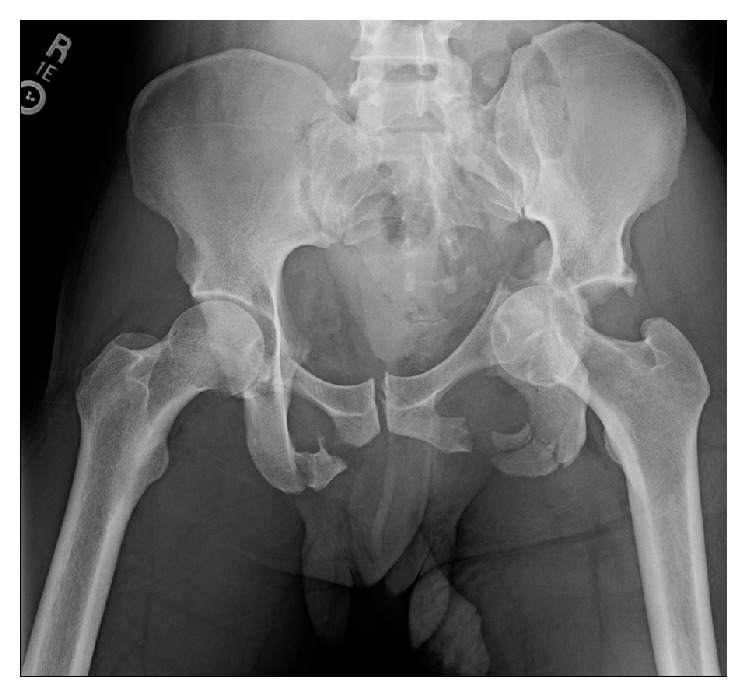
Trauma series AP pelvis radiograph.
